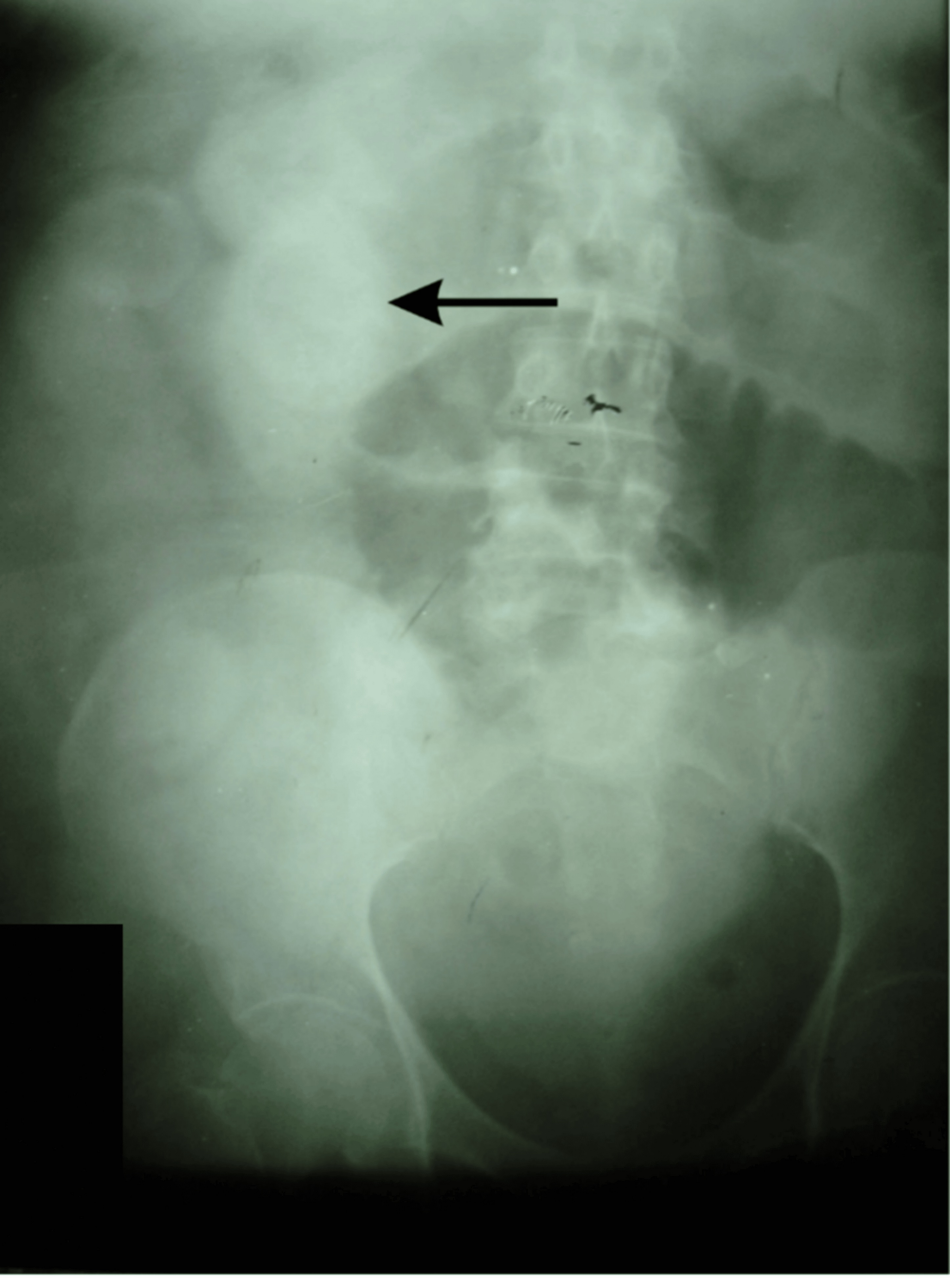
X-ray of the abdomen shows a calcified shadow on the right side of the abdomen.
